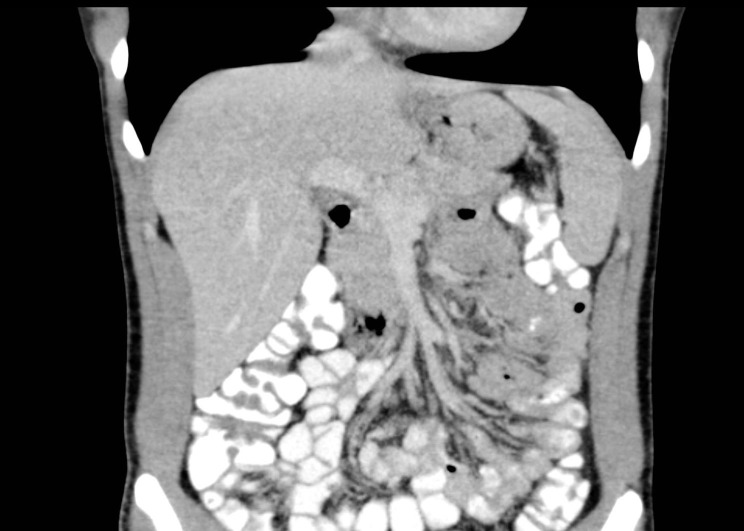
Coronal CT image with intravenous and oral contrast showing interval resolution of pneumatosis and portal venous gas.
